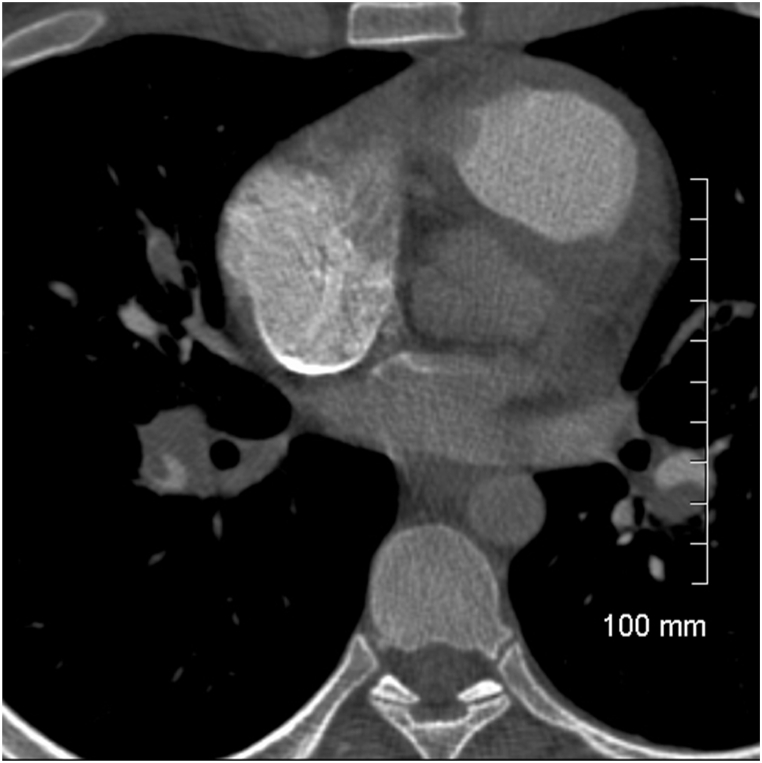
CT pulmonary angiogram demonstrating bilateral pulmonary emboli with contrast present in the left atrium suggesting PFO.
